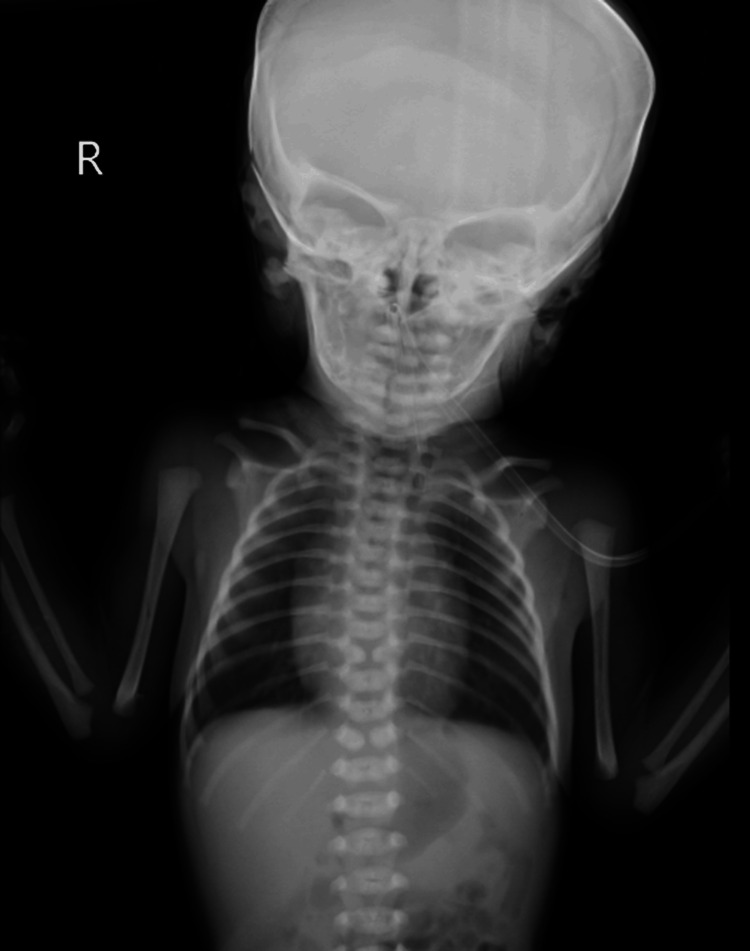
Postoperatively, X-ray imaging confirmed the successful separation of the trachea from the oesophagus
